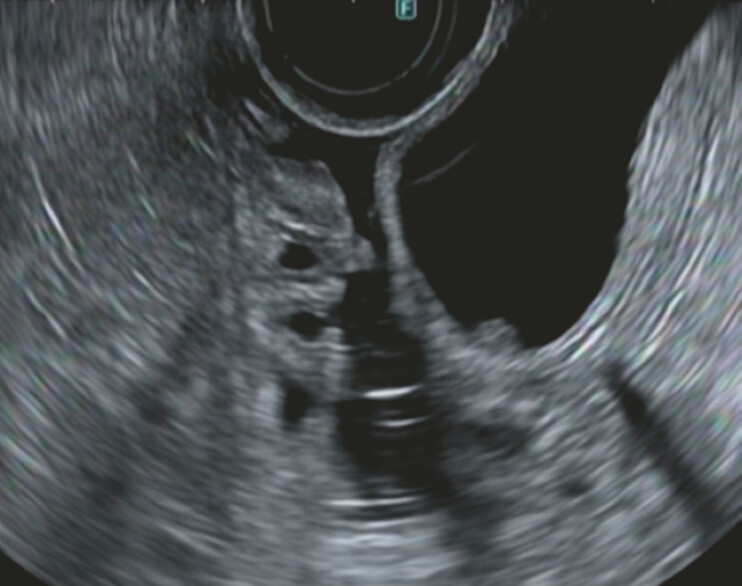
Endoscopic ultrasonography showed nodules in the choledochal cyst.
